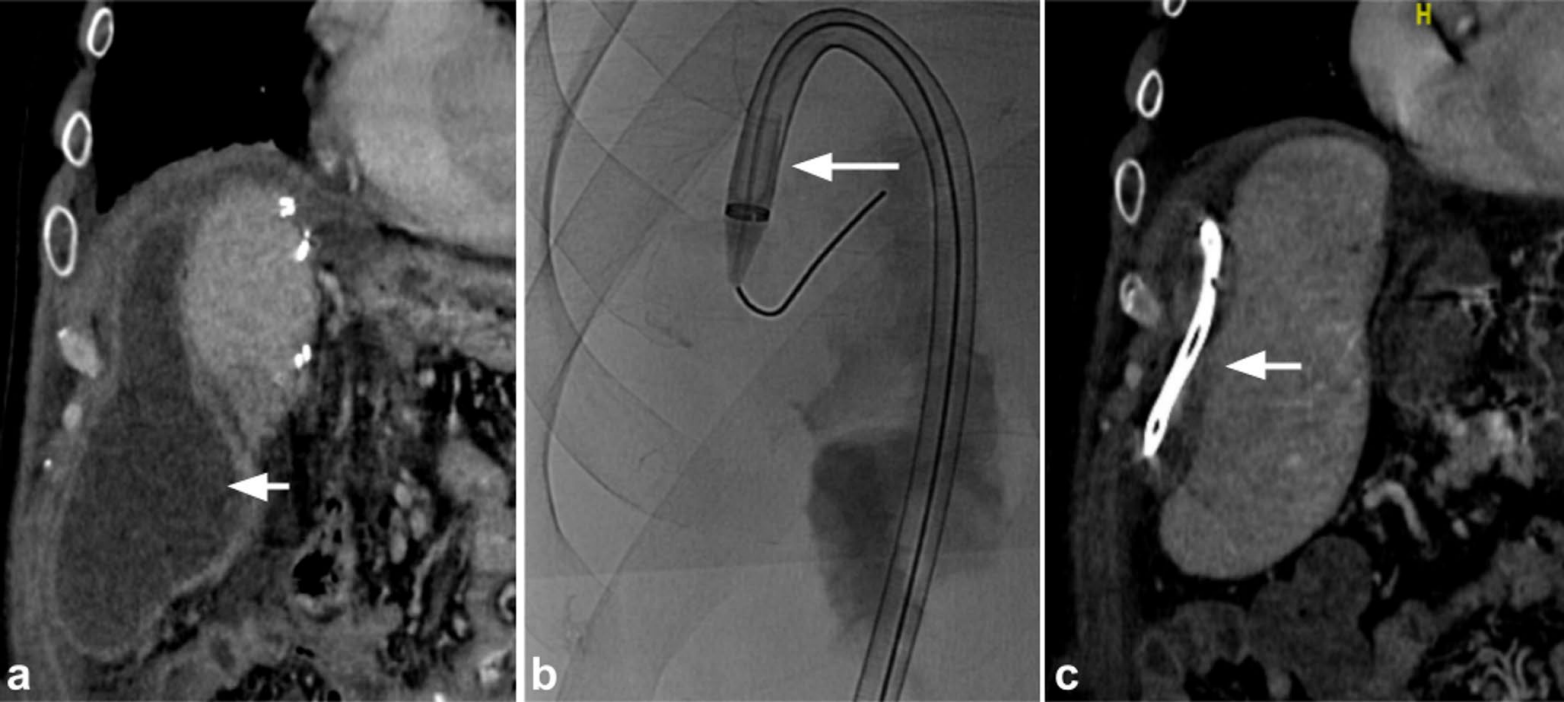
Large-bore aspiration of a postoperative intra-abdominal abscess using the FlowTriever Aspiration Catheter (a) Coronal contrast-enhanced computed tomography (CT) of the abdomen shows a 17.3 × 6.6 cm complex fluid collection following appendectomy for perforated appendicitis (arrow) (b) Fluoroscopic image shows the T20 FlowTriever Aspiration Catheter advanced into the collection (arrow) (c) Follow-up coronal contrast-enhanced CT of the abdomen and pelvis obtained one day later shows near-complete collapse of the cavity around the indwelling drain (arrow)
